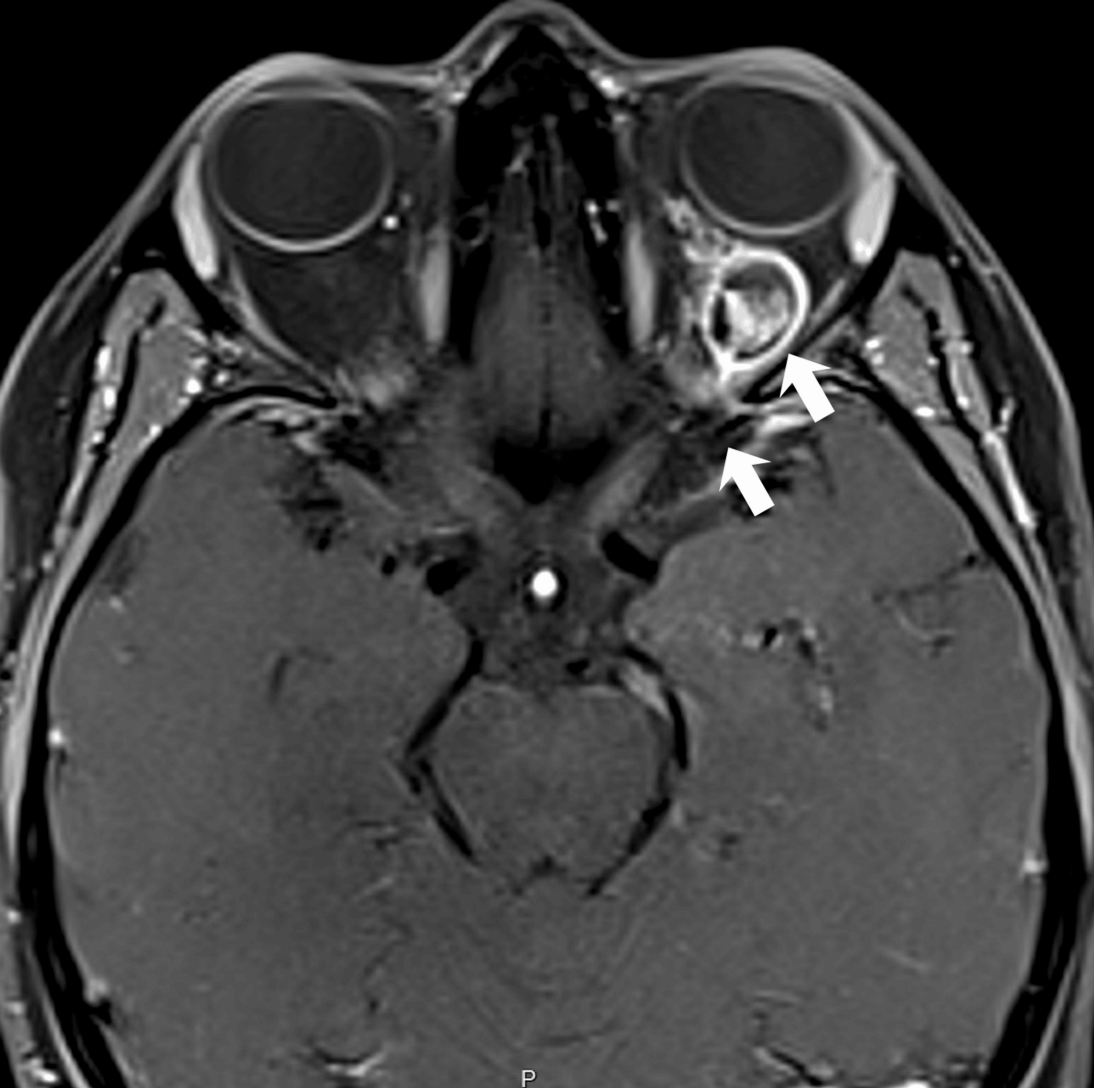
Retro-orbital cavernoma and venous extension 3D T1-weighted gradient echo (GRE) contrast-enhanced image of an intraconal, retro-orbital heterogeneous lesion containing hemoglobin degradation products, consistent with a cavernoma, associated with small ectatic vascular structures extending into the superficial medial cerebral veins through the superior orbital fissure.
